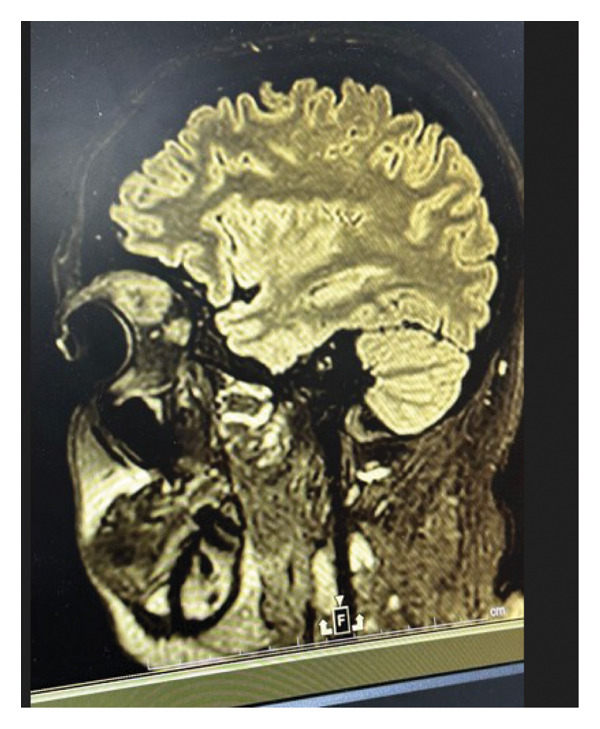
Sagittal T2‐weighted MRI showing a well‐defined orbital mass in the superior extraconal compartment of the left orbit.
